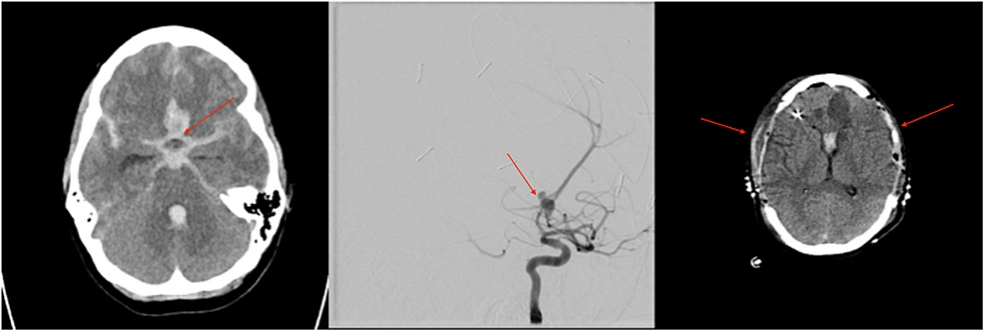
Imaging related to the clinical course of patient 3. (A) Head CT obtained on presentation demonstrated SAH secondary to a ruptured anterior communicating artery aneurysm. (B) Angiogram obtained after presentation demonstrating an anterior communicating artery aneurysm. (C) Post-operative head CT after bilateral DCHC for refractory elevated ICP. SAH, subarachnoid hemorrhage; DCHC, decompressive hemicraniectomy; ICP, intracranial pressure
